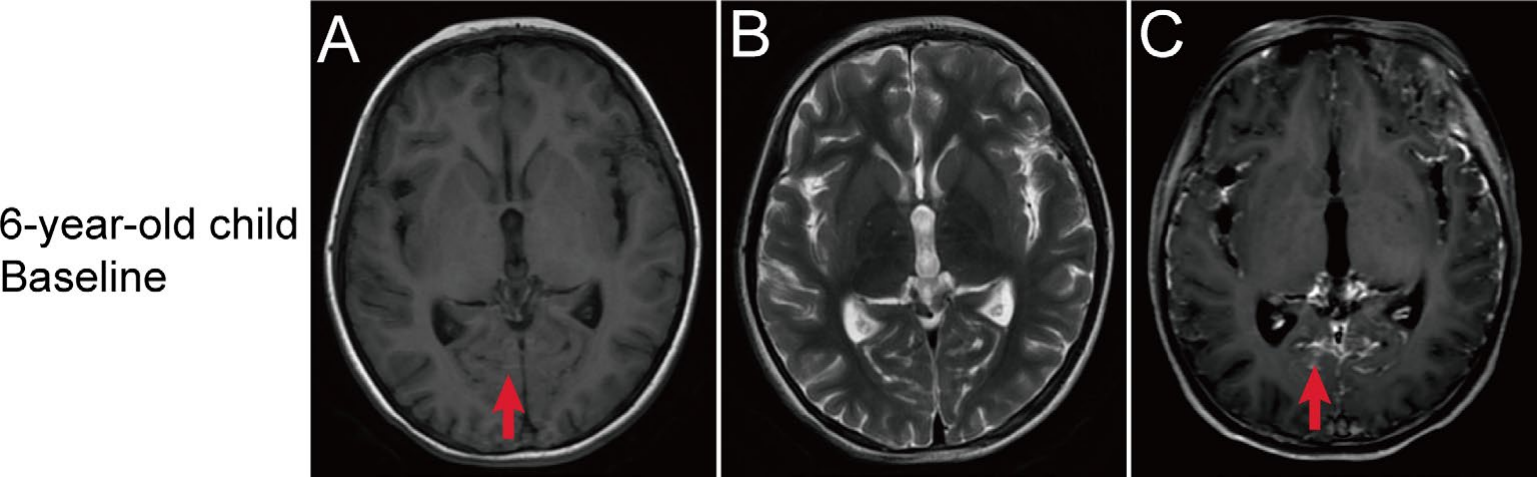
(A) Brain MRI at 6 years of age after the onset of symptoms reveals slightly hyperintense signals in the calcarine sulcus on T1WI. (B) Isointense signals in the calcarine sulcus on T2‐weighted images (T2WI). (C) Enhancement within the cerebral sulci and along the extensive leptomeninges on T1WI.
